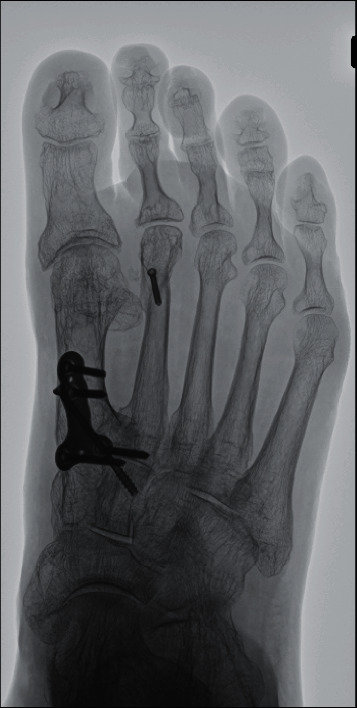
Radiograph one year postoperatively. The hallux valgus angle was 4° and the intermetatarsal angle 8°.
